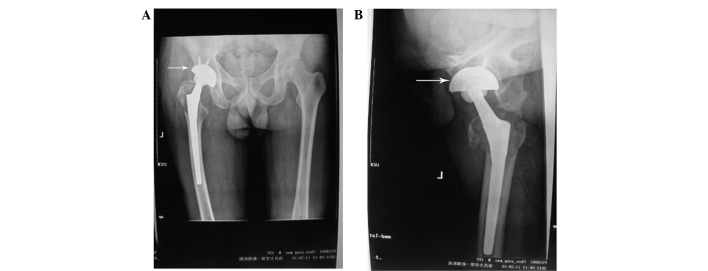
Post-operative (A) frontal and (B) lateral radiograph following the total hip arthroplasty (THA) of the left hip showing that the position of the the artificial joint prosthesis is stable (white arrow).
